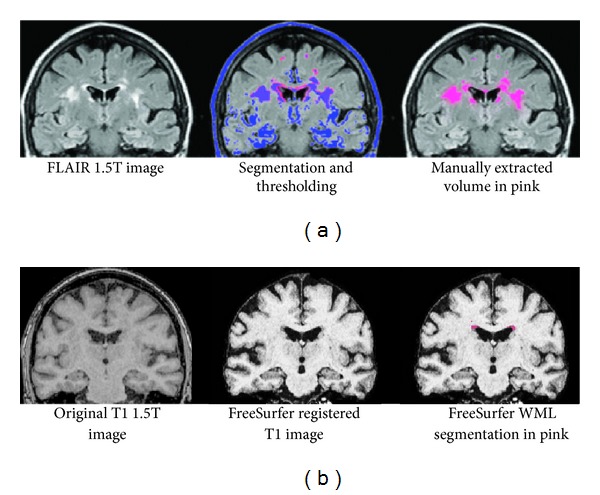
1.5T MR images from a patient with severe-burden WML showing the differences in scan sequence and WML detection between manual MRIcron volumetry (a) and automatic FreeSurfer volumetry (b).
